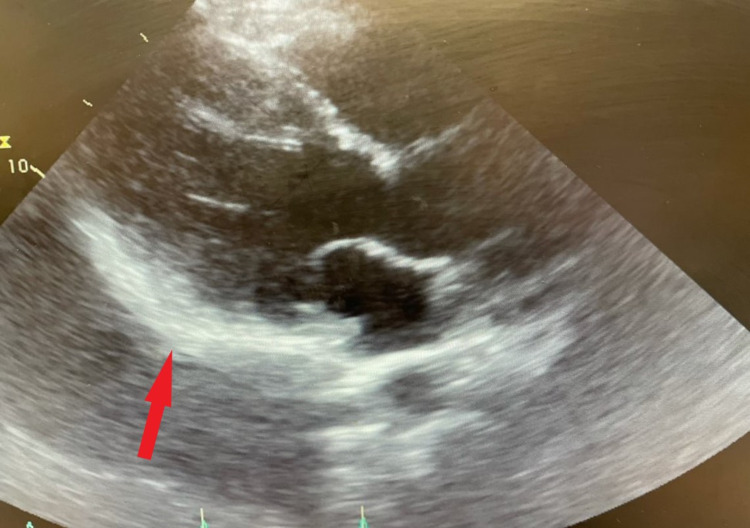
Echocardiogram showing epicardial brightness (red arrow) suggestive of pericarditis.
